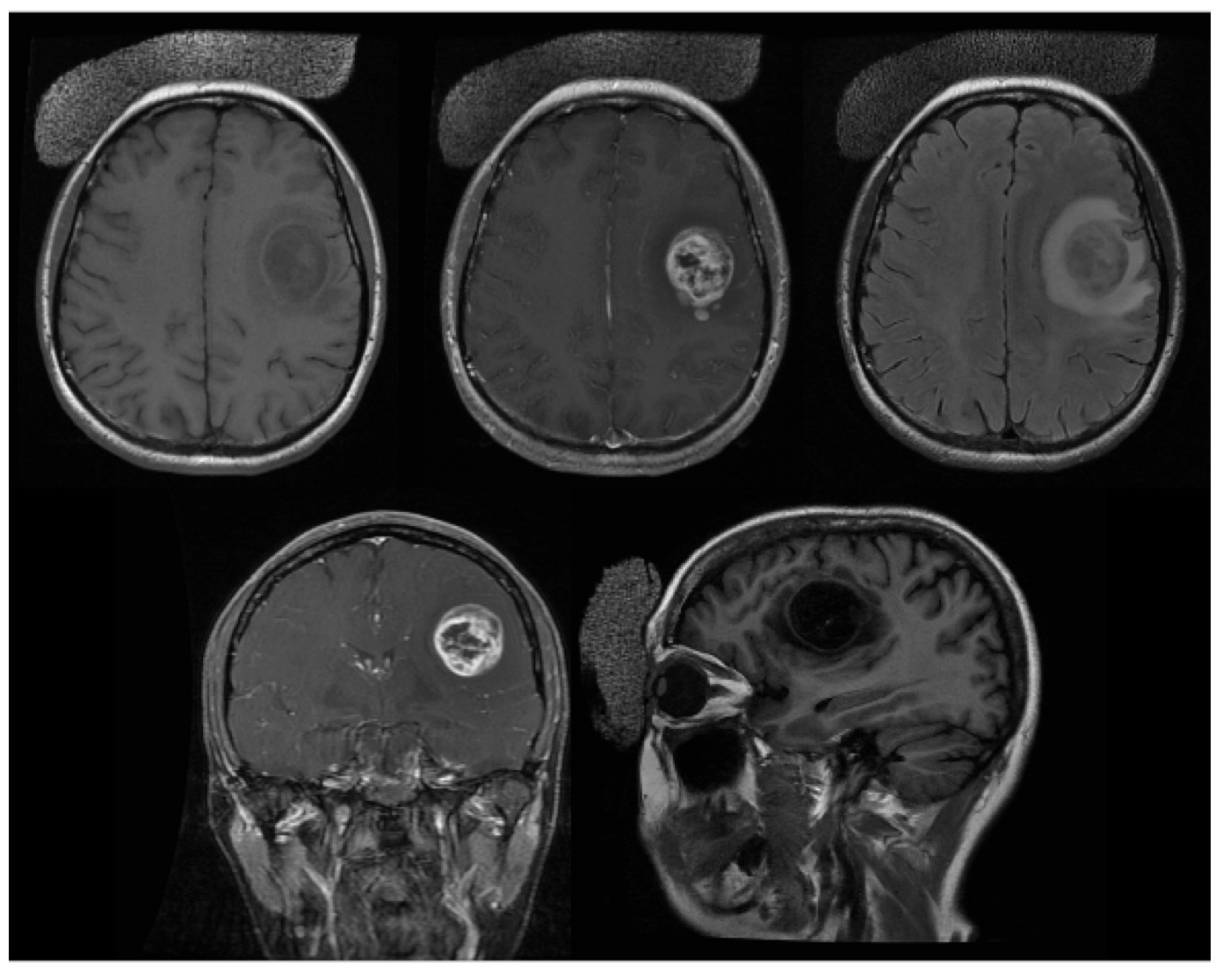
Preoperative Brain MRI Brain MRI shows left frontotemporal mass and T2/FLAIR hyperintensity
